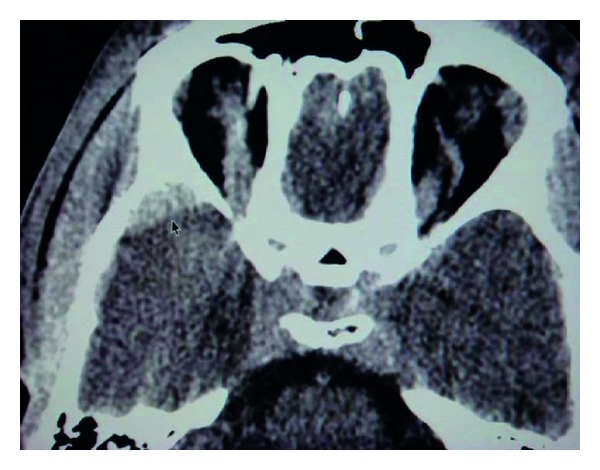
Computed tomography scan showing a small acute EDH in the right temporal region adjacent to the fracture.
